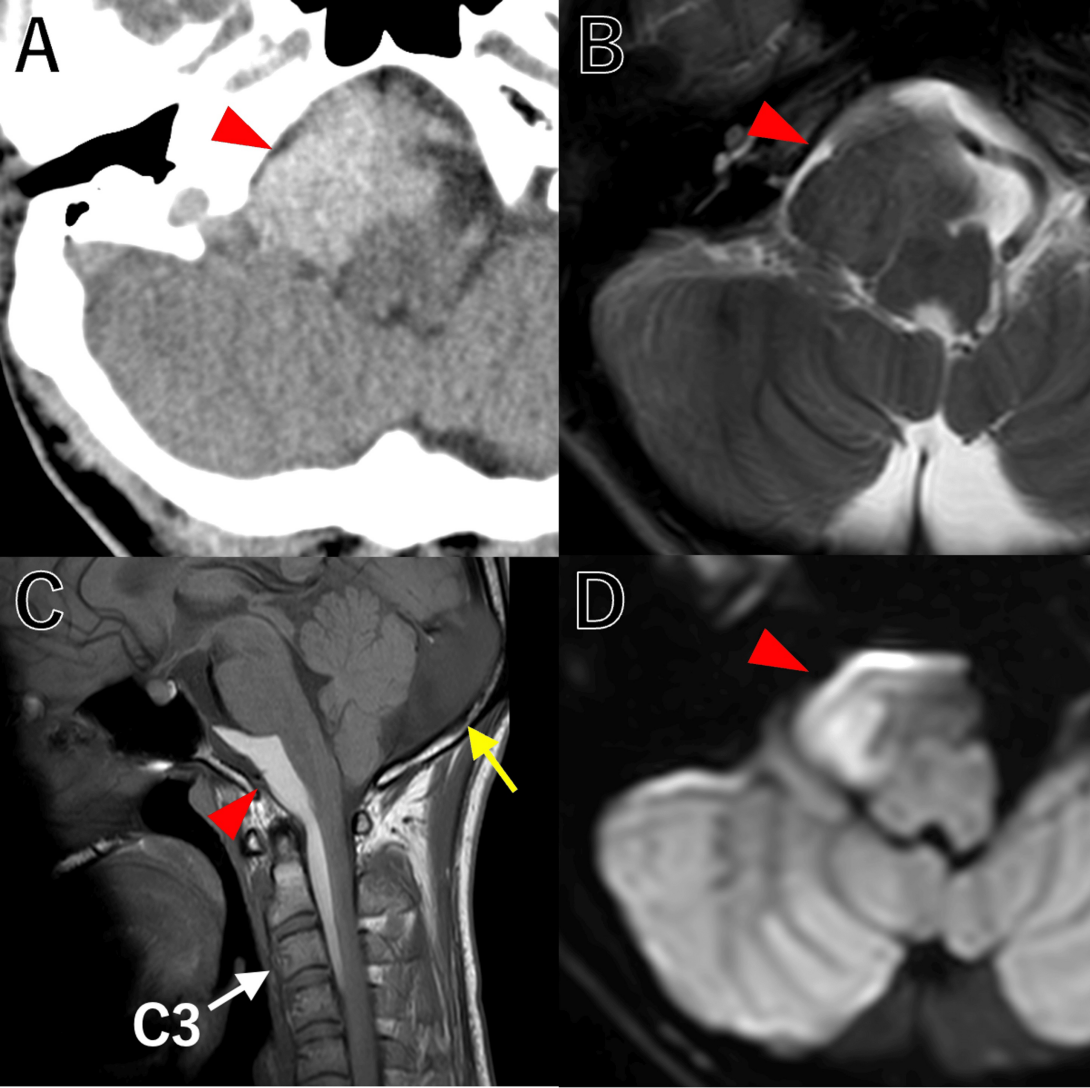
CT and MRI findings. Plain CT showed a high-density lesion (red arrowhead) in the right lateral medullary cistern adjacent to the jugular foramen (A). MRI showed a lesion (red arrowhead) with a T2 isointense signal (B), a T1 hyperintense signal (C), and DWI mixed intensity (D) extending from the posterior cranial fossa. The tumor extended into the cervical spinal canal (White arrow: the C3 vertebral body). A subtentorial arachnoid cyst (yellow arrow) was also present.
